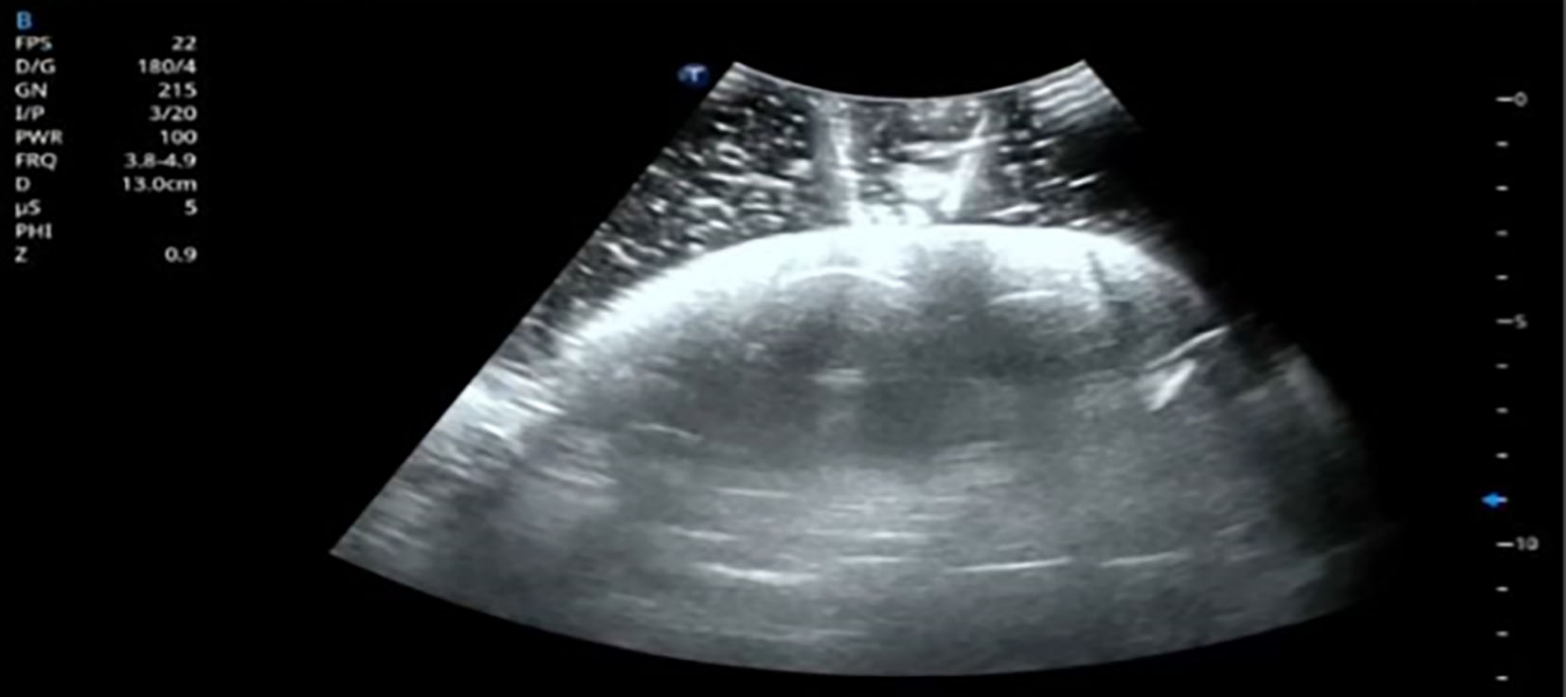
Ultrasound visualization of needle puncture through a silicone kidney model.
